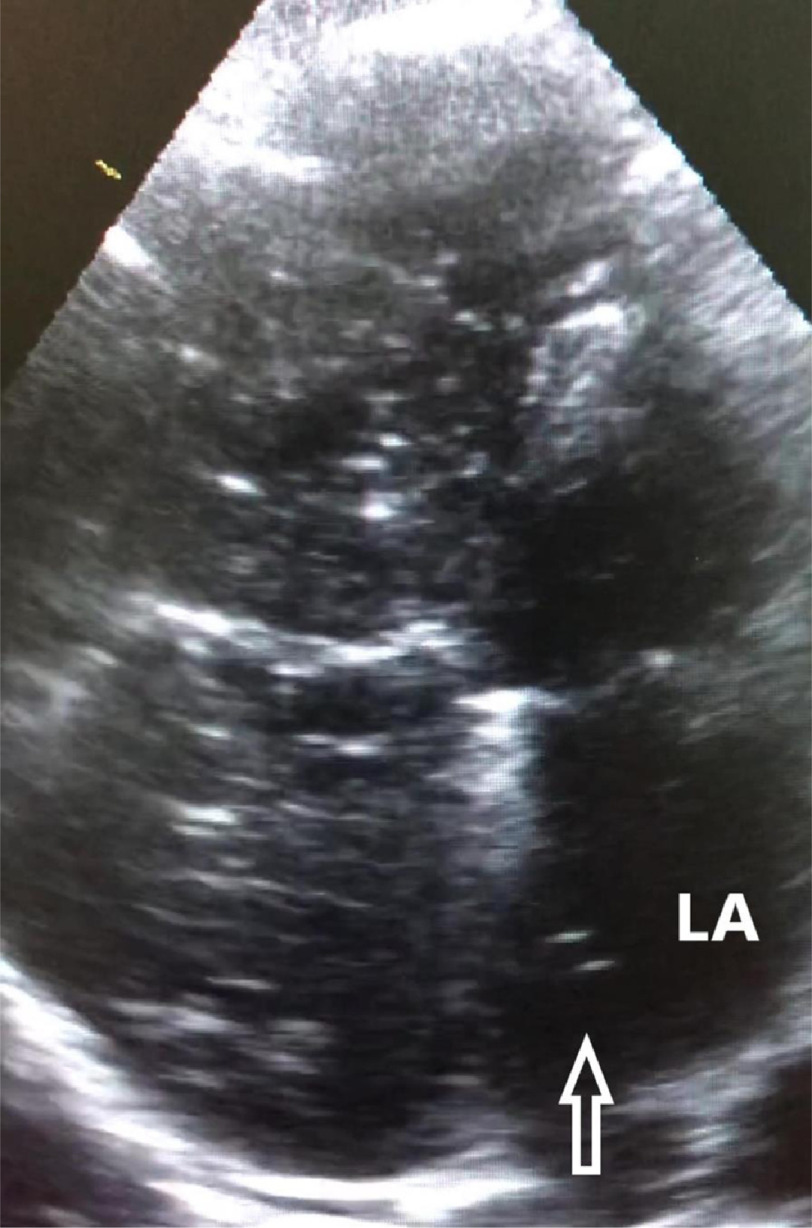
TTE using bubble contrast. Appearance of microbubbles (arrow) in left atrium (LA) in apical 4-chamber view on TTE is indicative of right to left atrial shunt.
